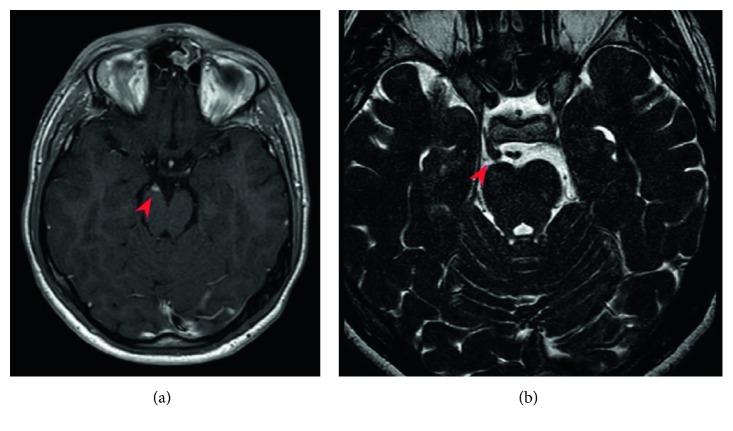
Axial T1-weighted (a) and axial T2-weighted (b) MRI images showing 5-6 mm nodular mass located within the fork of the right basilar artery near to perimesencephalic and interpeduncular cisterns (Oct 2018).
